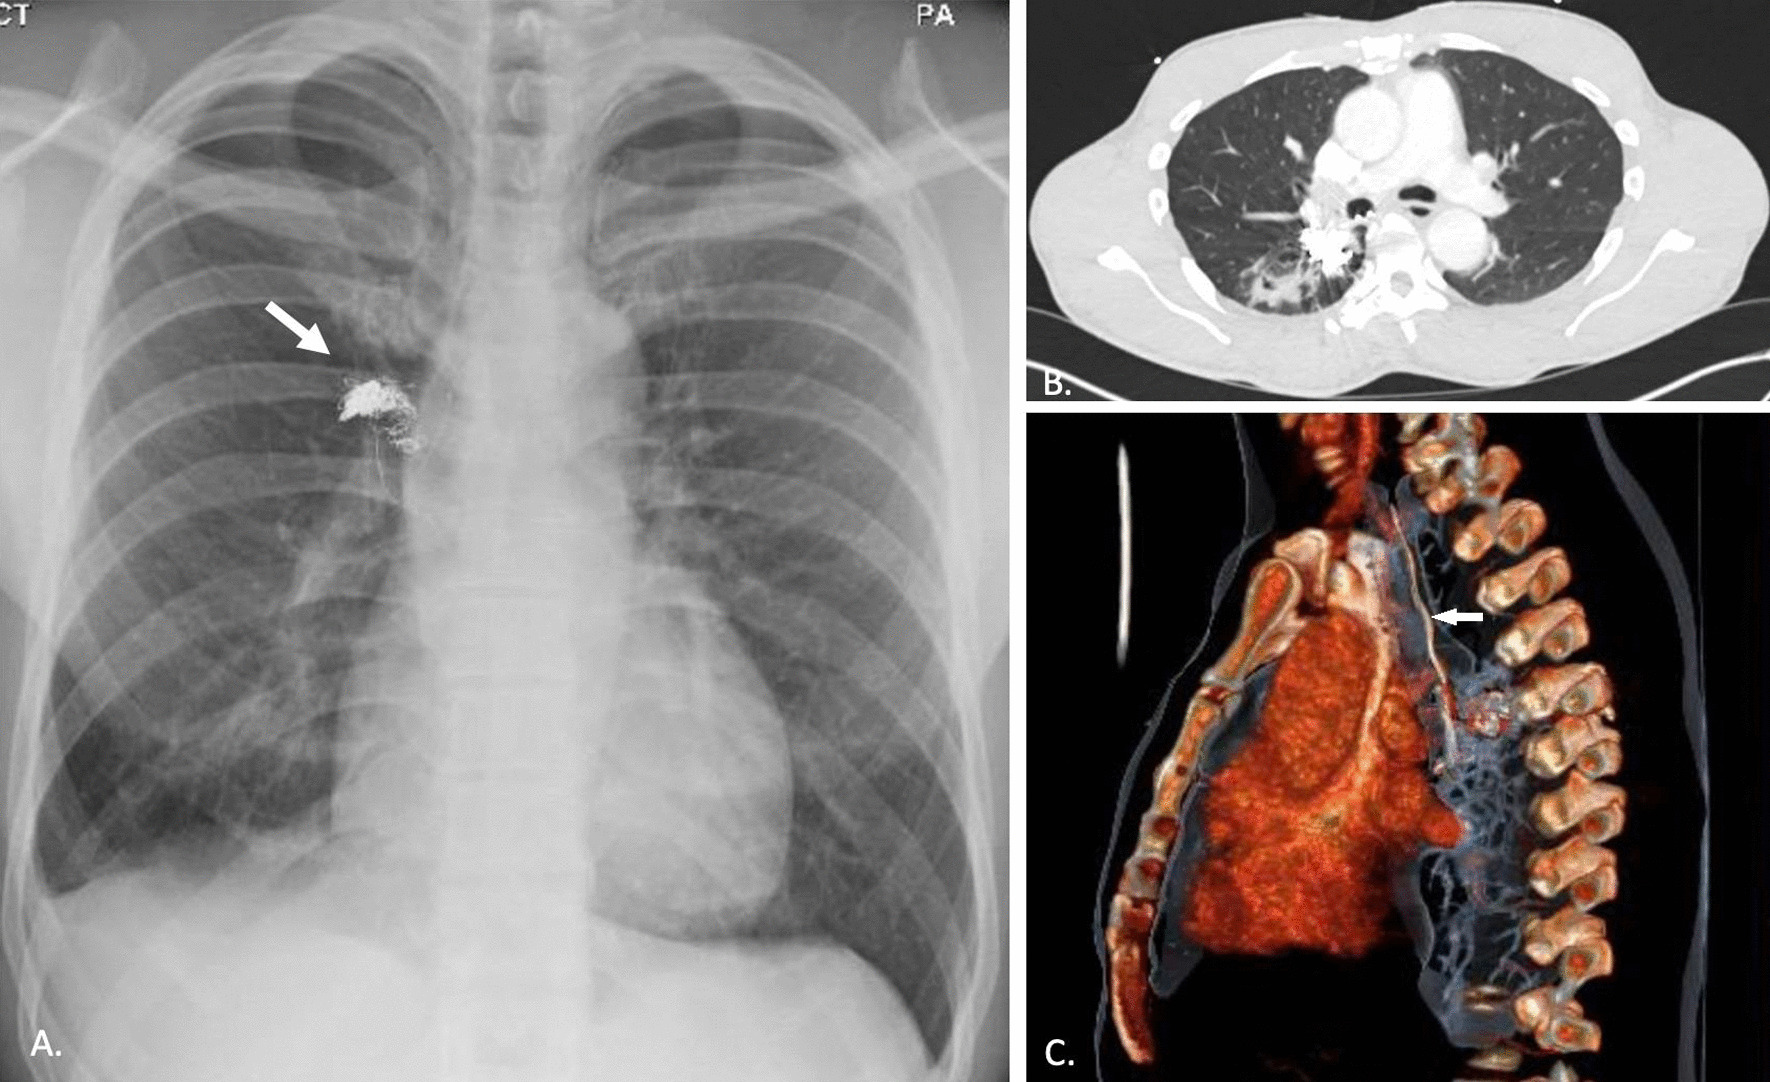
Radiological findings: A Chest x-ray showing the coils in the right chest (arrow). B Axial view confirming bunch of coils lying posterior to the hilum. Associated changes in the lung parenchyma are visible. C Reconstructed CT scan images demonstrating a coil extending into right bronchus and up to the larynx (arrow)
